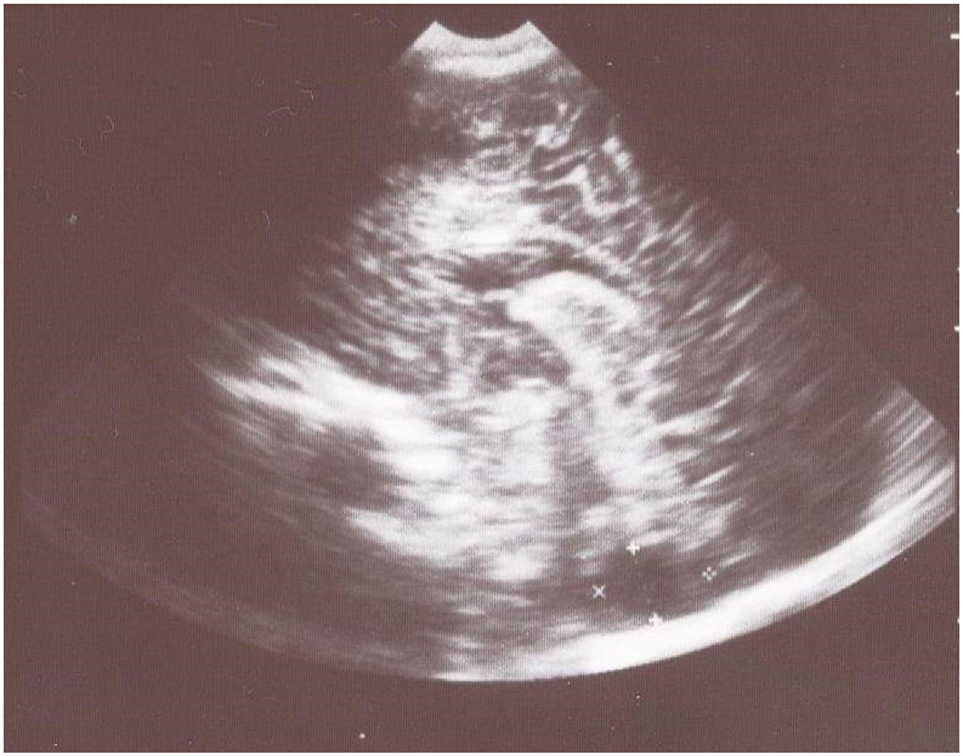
Transfontanelle sonography shows large cyst-like cisterna magna that suggested cerebellar hypoplasia and slightly dilated frontal horns of the lateral ventricles.
